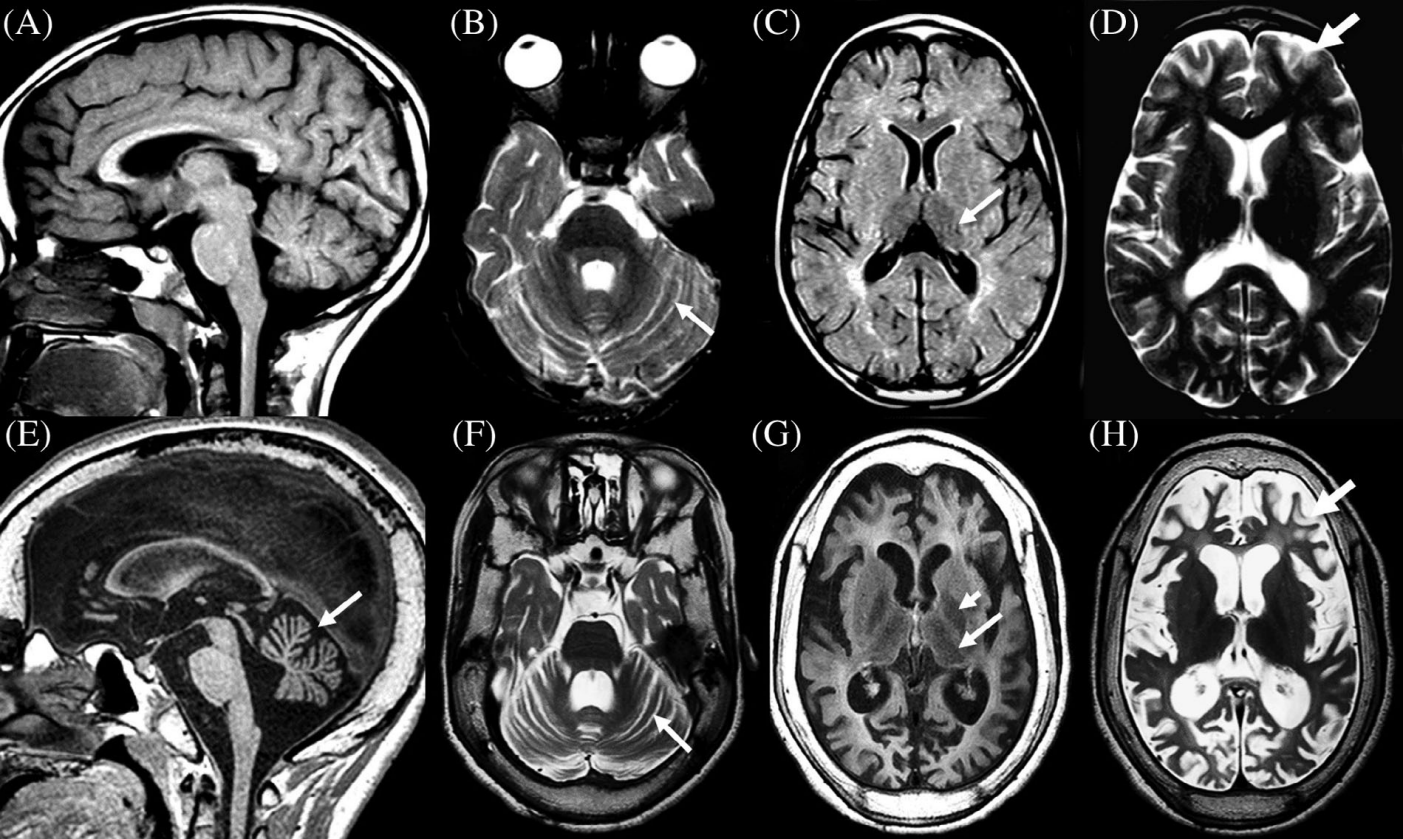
Two brain MRIs and disease progression in a patient with *CLN1 (PPT1*) associated disease (patient 5, ID#76 in Table 1). Top row 4y2m (A‐D): Sagittal T1 weighted image (A) demonstrates a normal corpus callosum, brainstem and vermis. Axial T2 weighted image (B) reveals mild widening of the interfoliate sulci (arrow) of the cerebellar hemispheres. Axial FLAIR image (C) shows dark signal intensity of the thalami (arrow) and increased signal of the periatrial white matter. Mild widening of the supratentorial sulci is noted (arrow) on T2 weighted axial image (D). Bottom row 7y8m (E‐H): Marked thickening of the calvarium is shown on all images. There is volume loss of the corpus callosum and moderate atrophy of the vermis (arrow) on T1 weighted sagittal image (E). Progressive widening of the interfoliate sulci (arrow) of the cerebellar hemispheres is shown on axial T2 weighted image (F). There is persistent loss of thalamic signal and volume (arrow) on axial FLAIR image (G). On the same image, loss of globus pallidus signal is present (short arrow) and the white matter is diffusely abnormally increased in signal. Marked widening of the supratentorial sulci (arrow) and moderate ventricular enlargement is noted on axial T2 weighted image (H), reflecting both central and peripheral volume loss
